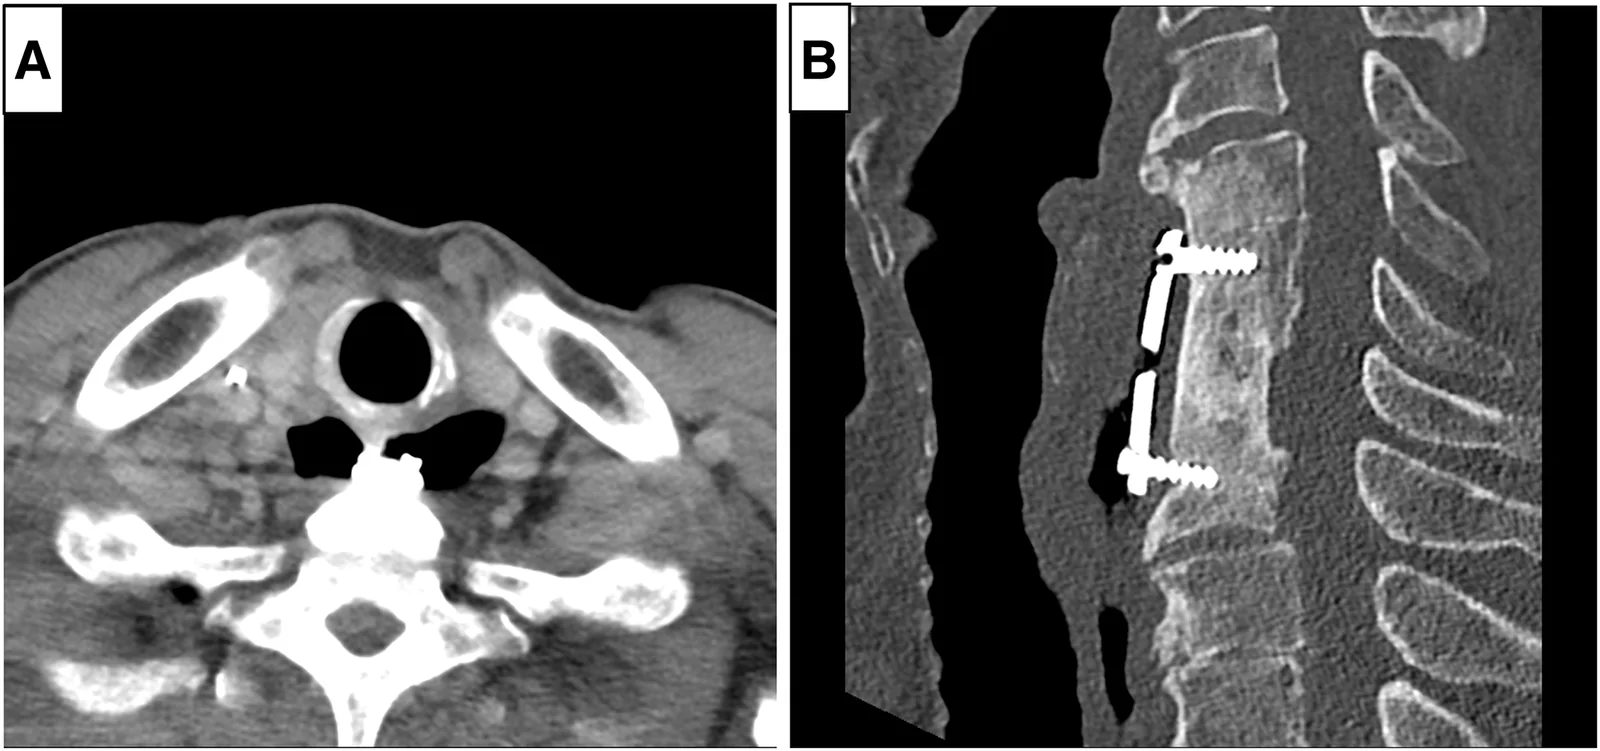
CT examination. (A) A metallic plate is present in the dilated cervical esophagus. (B) The 5th to 7th cervical spine was fixed by bone screwing with a metallic plate.
